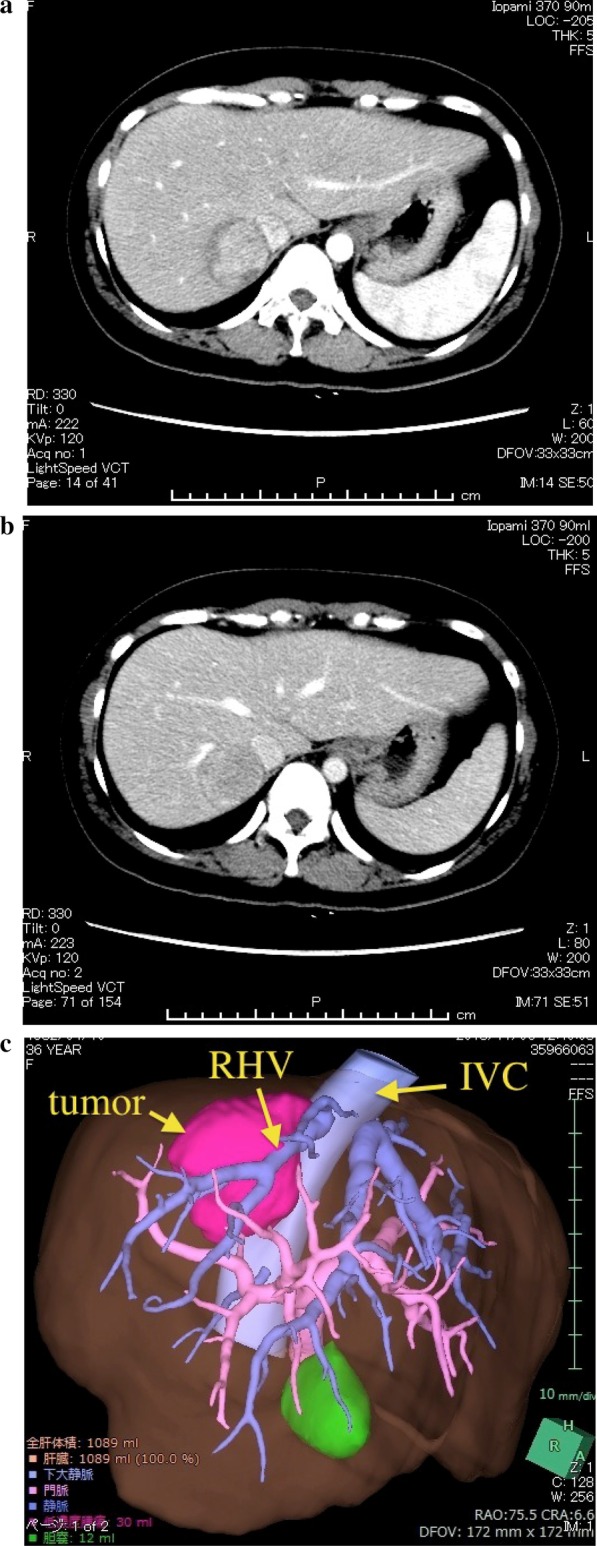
Abdominal contrast-enhanced computed tomography revealing a 40-mm tumor, which was a well enhanced in the arterial phase and b washed out in the portal venous and delayed phases, suggesting hepatocellular carcinoma recurrence. The three-dimensional image (VINCENT) showed the tumor adjacent to the inferior vena cava and right hepatic vein (c)
